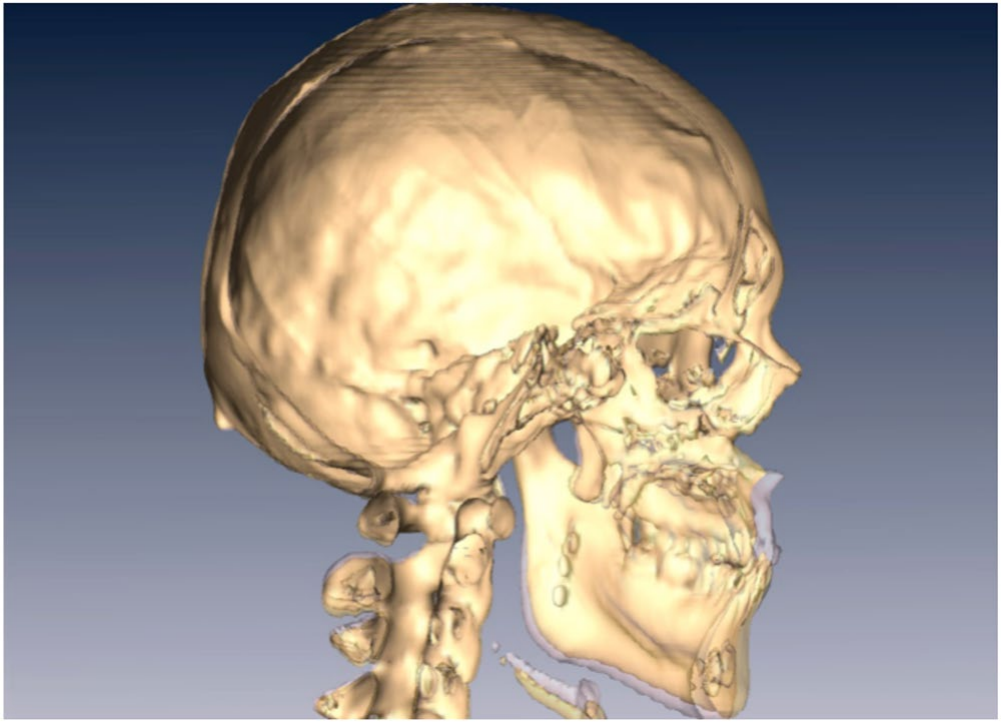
Superimposition of three dimensional CT scans before (yellow) and six months after surgery (light purple). Notice the maxilla has been advanced and the mandible has been moved posteriorly. There is also clockwise rotation of the entire maxilla-mandible complex.
